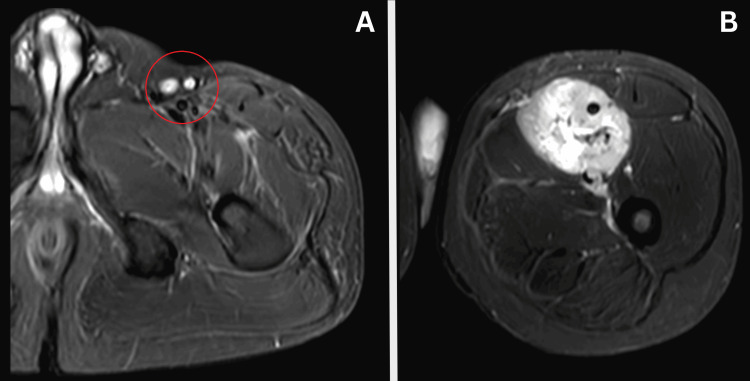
Magnetic resonance imaging (MRI) of the left thigh showing short tau inversion recovery (STIR) axial images of the swelling at different levels. A) Femoral artery, femoral vein, and femoral nerve (indicated by a red circle); B) At the inferior level, the mass is originating from the femoral vein, so the vein is not visible as it was in image A, and the lesion is encasing the femoral artery.
